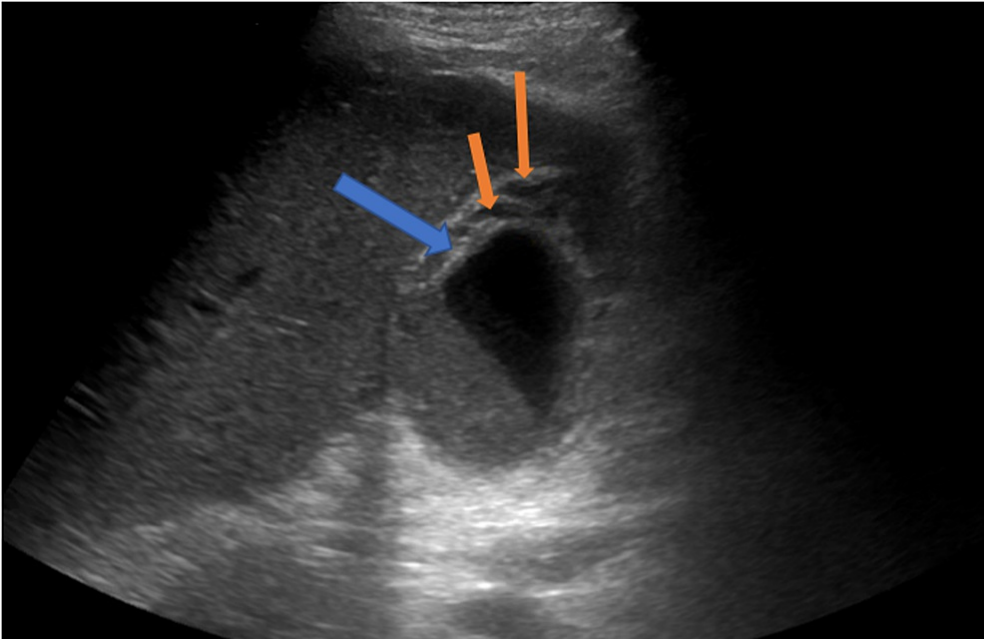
Ultrasound (trans gallbladder view) of the gallbladder consistent with acute cholecystitis. Thickened gallbladder wall (blue arrow) and pericholecystic fluid (orange arrows).
